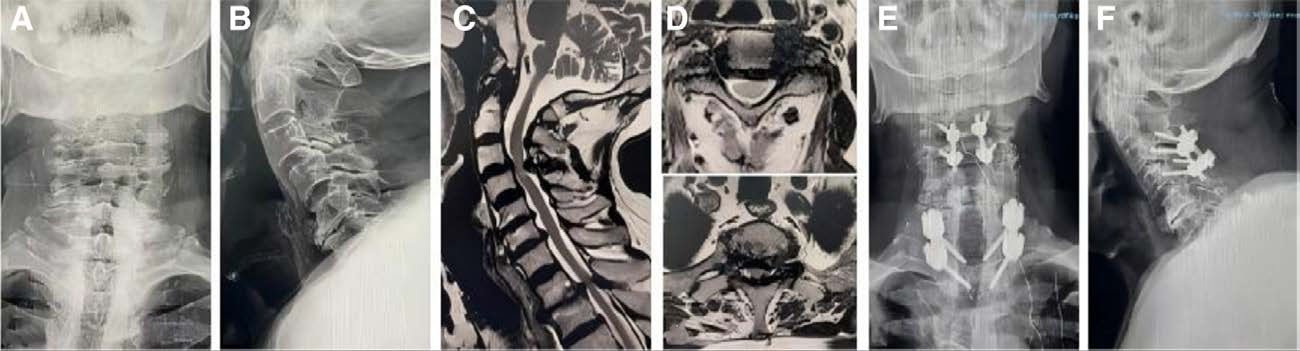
Case 2: (A and B) cervical X-ray shows cervical degeneration, osteophyte with intervertebral space stenosis; (C and D) MRI of cervical spine showed protruded intervertebral disc in C3/4, T1/T2 compression of the spinal cord; (E and F) postoperative X-ray images of jumping cervical spine posterior decompression and lateral block screw fixation suggest good internal fixation position.
